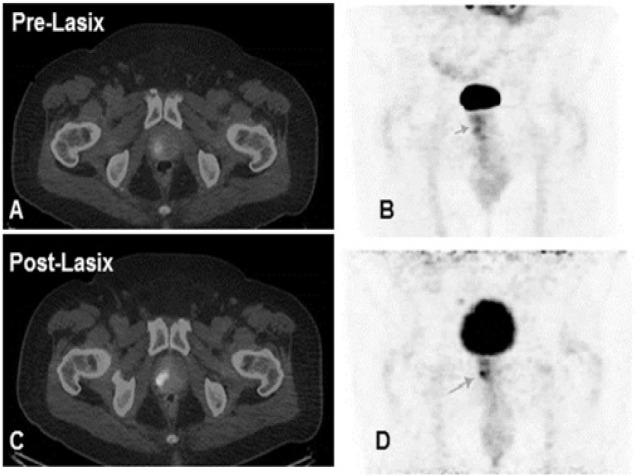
Fused PET/CT Images (A) and MIP (B) Showing Ill-Defined Lesion (Arrows) in the Prostate Gland. Post Lasix study in (C-D) showing better delineation of the lesion due to improvement of the image contrast
